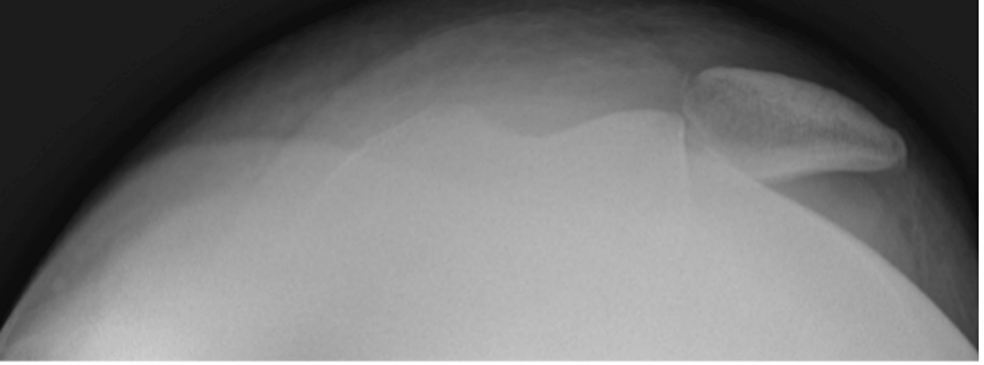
Modified sunrise (patient unable to bend the knee) radiograph of the left knee showing lateral patellar dislocation with medial patellar impaction defect and avulsion fracture of the medial patella
